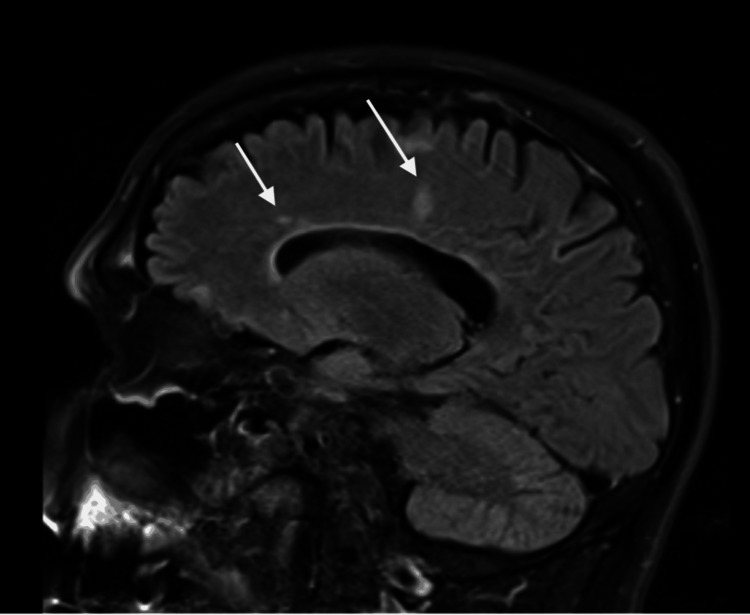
MRI brain showing findings consistent with MS but no evidence of metastasis. Arrows point to the findings consistent with multiple sclerosis (MS).
